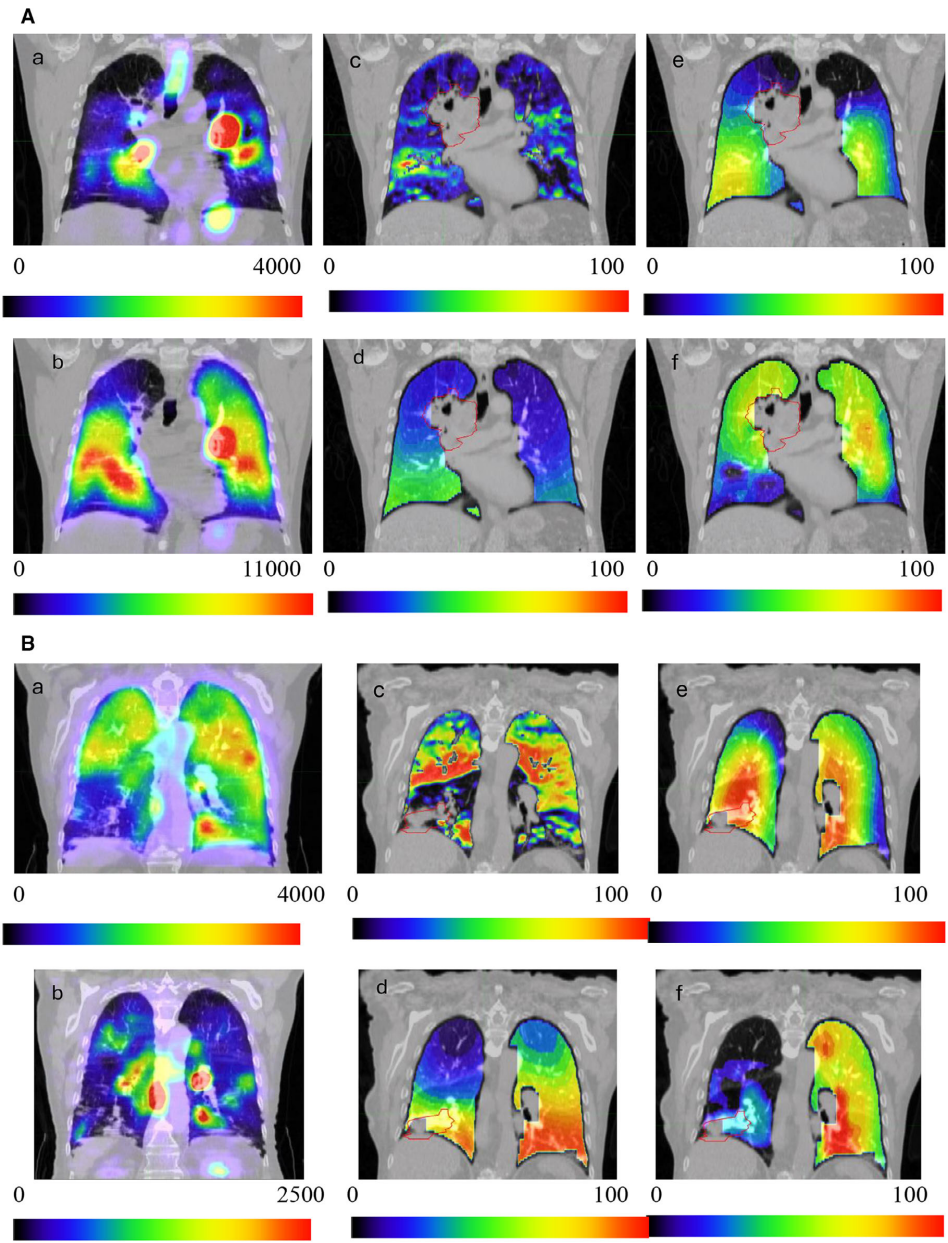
A. Representative examples of ventilation and perfusion images from the various functional imaging techniques investigated in this study. The red contour depicts the gross tumor volume (GTV). (a, and b) Ventilation and perfusion images, respectively, acquired using the standard‐of‐care SPECT technique. (c‐e) 4DCT‐ventilation images based on the Hounsfield Unit (4DCT‐vent‐HU, c), Integrated Jacobian Formulation (4DCT‐vent‐IJF, d), and Mass Conserving Volume Change (4DCT‐vent‐MCVC, e) techniques. (f) 4DCT‐based perfusion imaging (4DCT‐Perf). Figure 2‐B. Representative examples of ventilation and perfusion images from the various functional imaging techniques investigated in this study. The red contour depicts the gross tumor volume (GTV). (a, and b) Ventilation and perfusion images, respectively, acquired using the standard‐of‐care SPECT technique. (c‐e) 4DCT‐ventilation images based on the Hounsfield Unit (4DCT‐vent‐HU, c), Integrated Jacobian Formulation (4DCT‐vent‐IJF, d), and Mass Conserving Volume Change (4DCT‐vent‐MCVC, e) techniques. (f) 4DCT‐based perfusion imaging (4DCT‐Perf).
